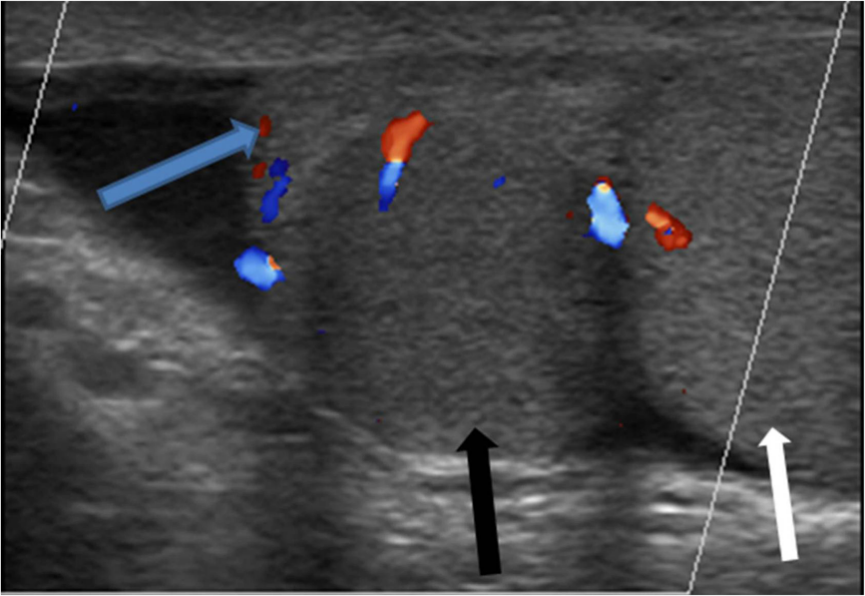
Ultrasound of patient 1. Longitudinal section of a color Doppler ultrasound shows normal testis (white arrow) in the right hemiscrotum and a 1.5cm oval mass in the right hemiscrotum (black arrow), adjacent to the upper pole of the right testis and slightly compressing the epididymis (blue arrow). This mass has the same echogenicity and normal flow as the testis nearby.
